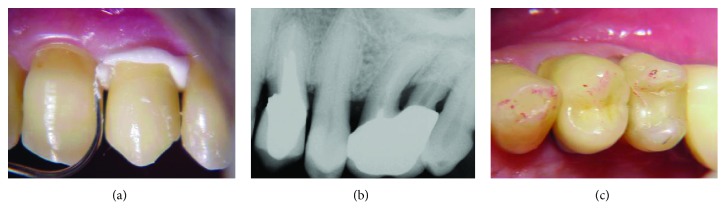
(a) Definitive cementation of prosthetic parts (core and crown); (b) final radiography; (c) verification and adjustments of occlusal contacts.
